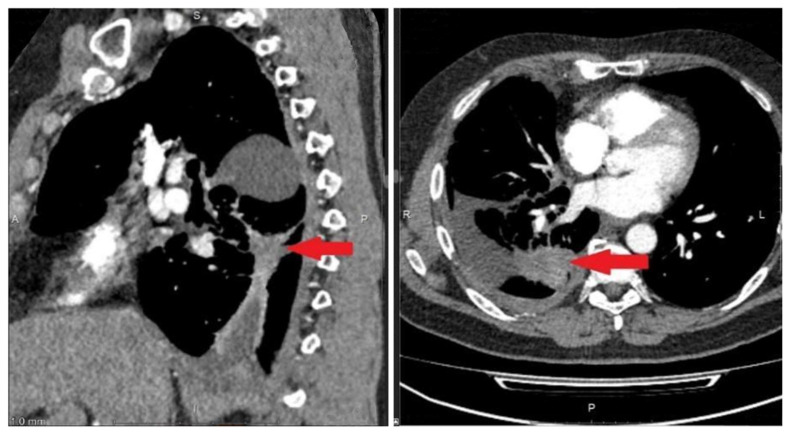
Protocol code 10: CT scan of the patient’s chest dated 19 February 2021. CT signs of fibroatelectasis S6 on the right, right-sided small hydrothorax, in comparison with the previous CT scan dated 17 December 2020, straightening of the lower lobe of the right lung is highlighted, a decrease in the amount of fluid in the right pleural cavity (indicated by arrow).
